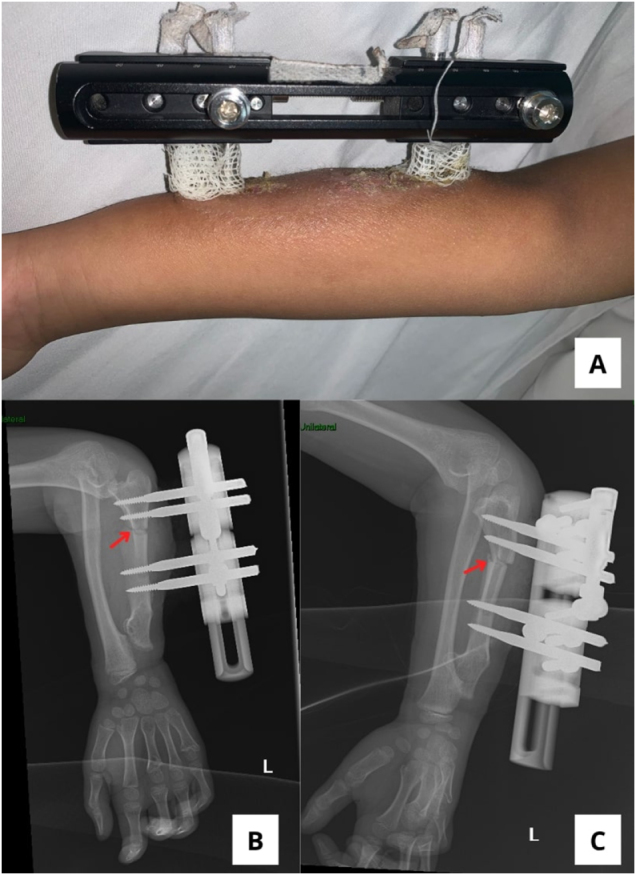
Immediately post-operative shows (A) LRS device was successfully applied. Plain radiograph of forearm (A) AP view and (C) lateral view shows signs of osteotomy (red arrow) and LRS devices on the left arm.
